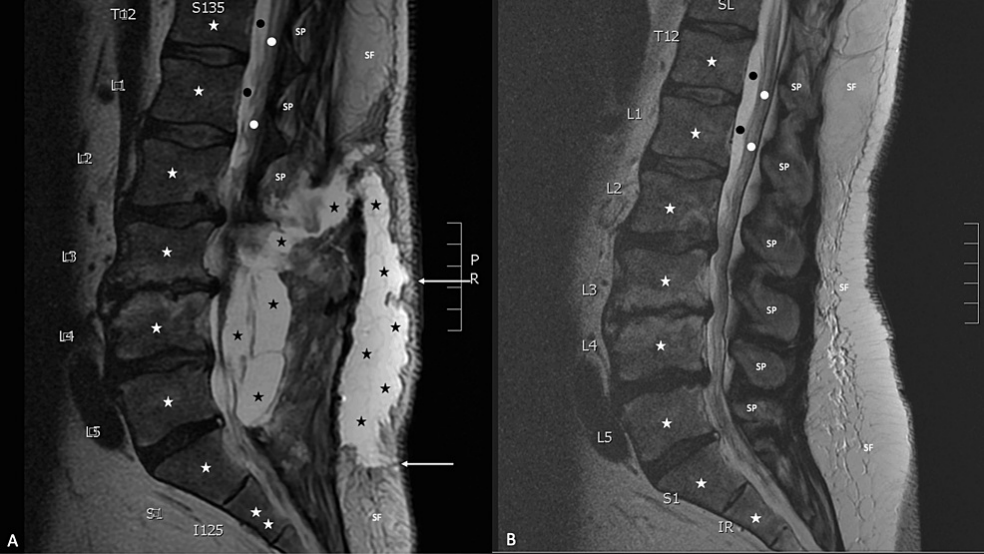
Post-surgical and pre-surgical sagittal views of magnetic resonance imaging with contrast of the lower back A magnetic resonance imaging of the lumber back of a 58-year-old man was performed on a 1.5 Tesla scanner, with sagittal short T1 inversion recovery, T1-weighted, and T2-weighted imaging 12 days after surgery to evaluated him for a post-operative cerebrospinal fluid leak (A). The sagittal view demonstrates the vertebral bodies (white stars) and spinal process (labeled SP) of the lower thoracic (labeled T12), lumbar (labeled L1 to L5) and upper sacral (labeled S1) vertebrae. The lower portion of the spinal cord (white dots) and cerebrospinal fluid in the dura (black dots) can also be seen. In addition, subcutaneous fat (labeled SF) can be noted. There are two large, connected collections of cerebrospinal fluid (black stars) in the soft tissue of the back; two tracts containing cerebrospinal fluid extend from the soft tissue collection to the skin surface (white arrows). The sagittal view of a similarly performed magnetic resonance imaging, from 18 months earlier, does not show any leakage of cerebrospinal fluid (B).
